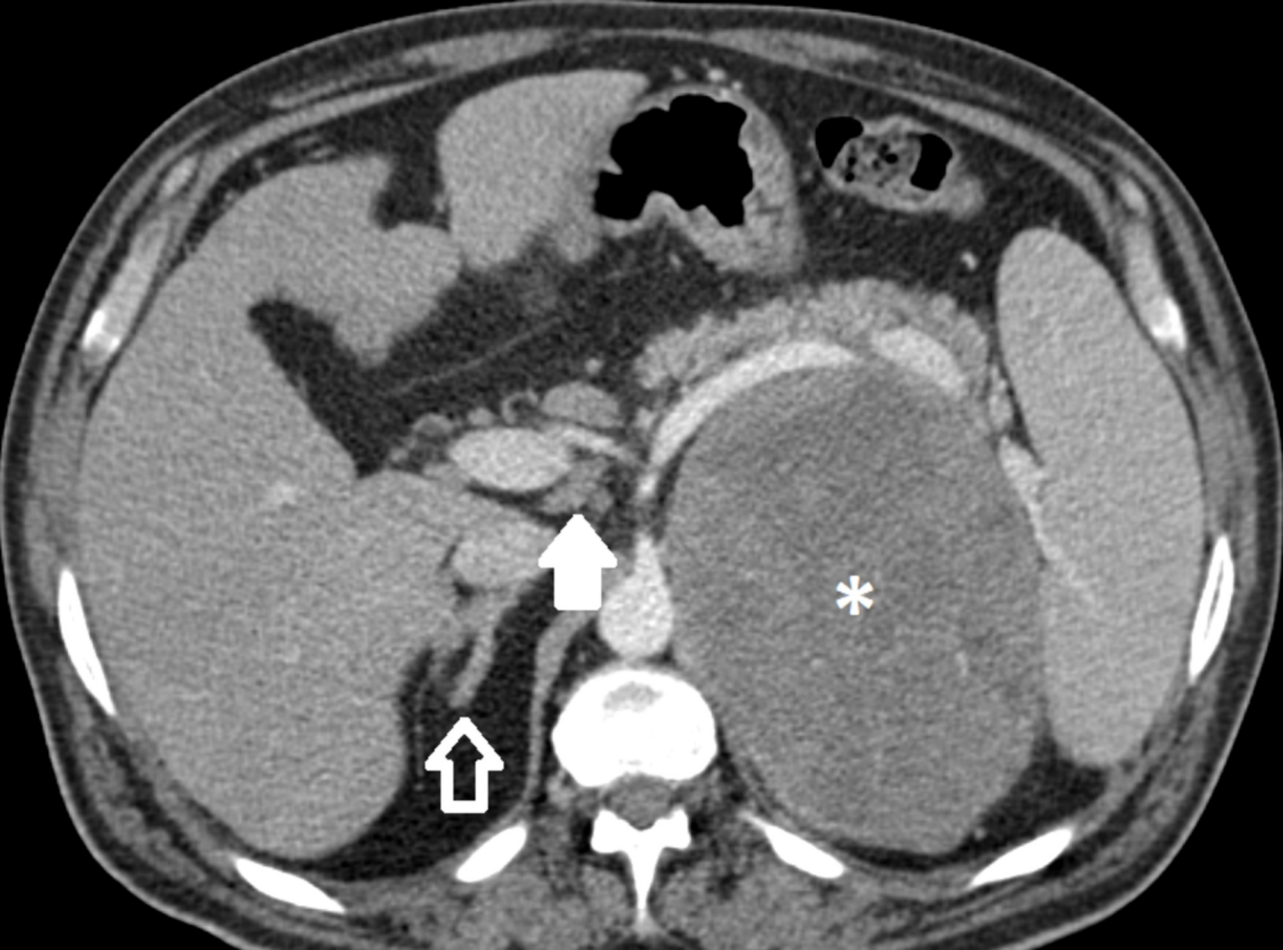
Contrast-enhanced computed tomography (CT) Axial images show a large adrenal mass (asterisk) with a focus of calcification (not shown) arising from the left adrenal gland, measuring higher than 10 Hounsfield units in density. There is local mass effect on the pancreas and spleen. The normal right adrenal gland is pictured for comparison (open arrow). A few sub-centimeter retroperitoneal lymph nodes are present (solid arrow).
